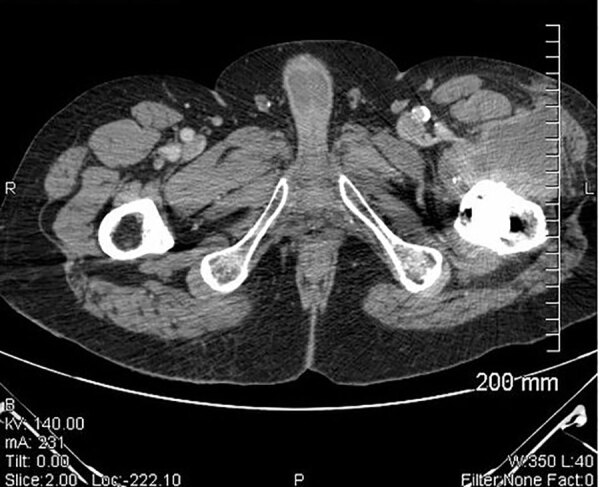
Computed tomography scan of pelvis in case 1 showing large abscess of the left hip with a periosteal image compatible with an osteitis in case of *Francisella tularensis*–related prosthetic joint infection, France.
